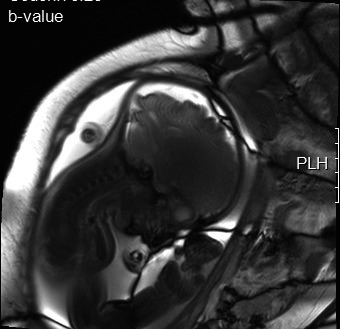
Prenatal fetal MRI at 32 + 2 wk of gestation
